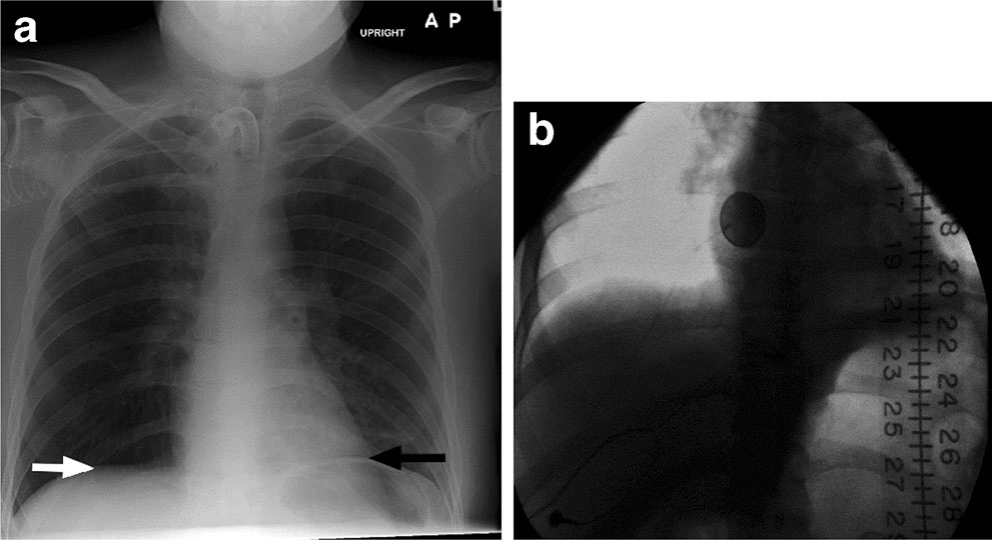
a Anteroposterior radiograph of a 29-year-old male with C2 tetraplegia from a diving accident shows elevation of the left hemidiaphragm (*black arrow*) in relation to the right (*white arrow*), concerning for diaphragmatic paralysis. b Fluoroscopic spot image from the subsequently obtained “sniff” test confirms the diagnosis
